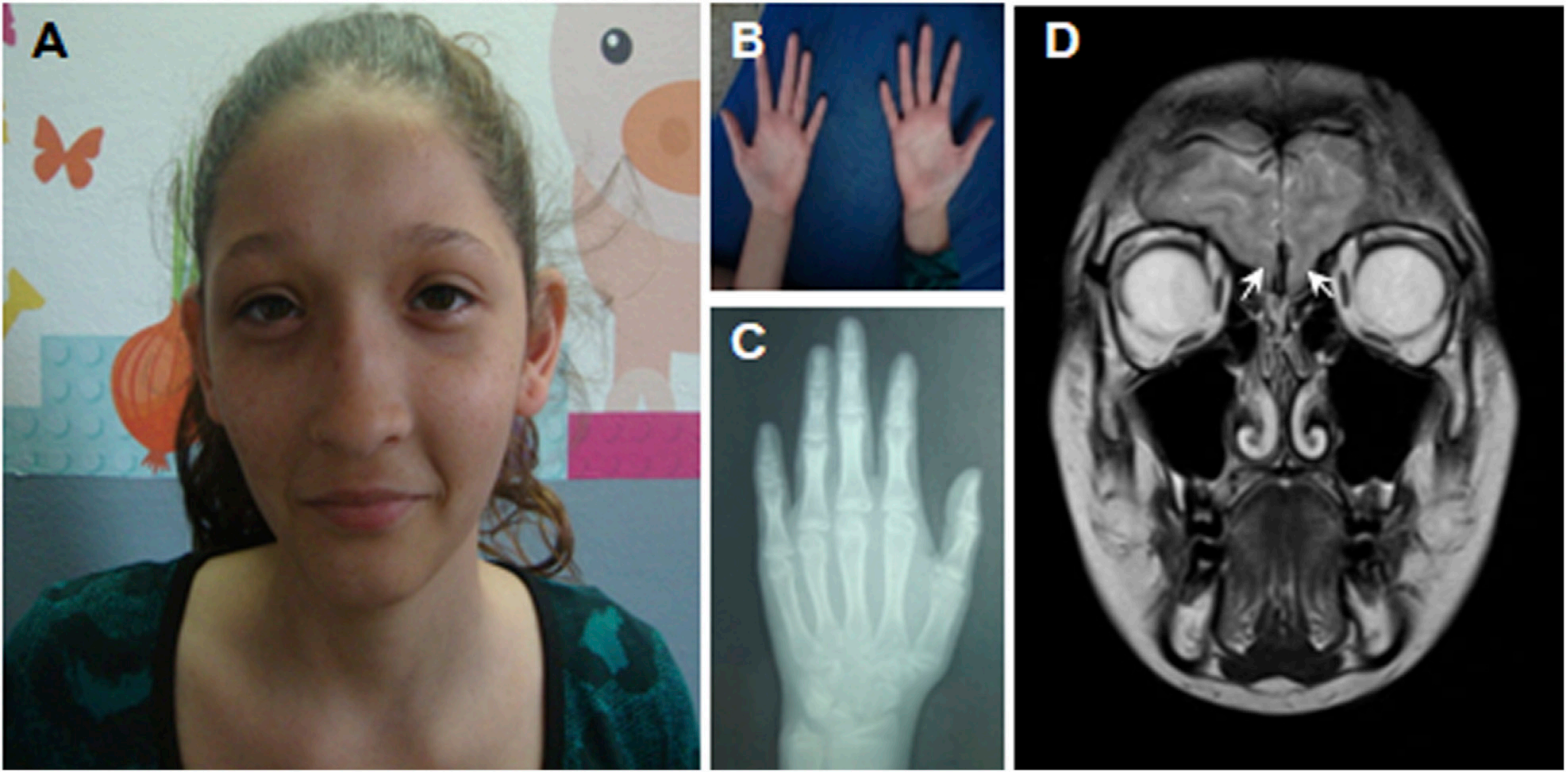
Anatomic features and bone age of the patient at the age of 14 years. (A) Microcephaly, long face with a high anterior hairline, broad forehead, epicanthal folds, strabismus, wide medial eyebrows, wide nasal base, prominent nasal bridge, short and smooth philtrum, small mouth, ears with overfolded helix, and large lobes. (B) Hands with thin digits and radial deviation of the second digits bilaterally, clinodactyly of the fifth finger. (C) Bone age was estimated between 12 and 13 years, according to Greulich and Pyle (1959). (D) MRI of the brain showing the absence of the olfactory bulbs (white arrows).
